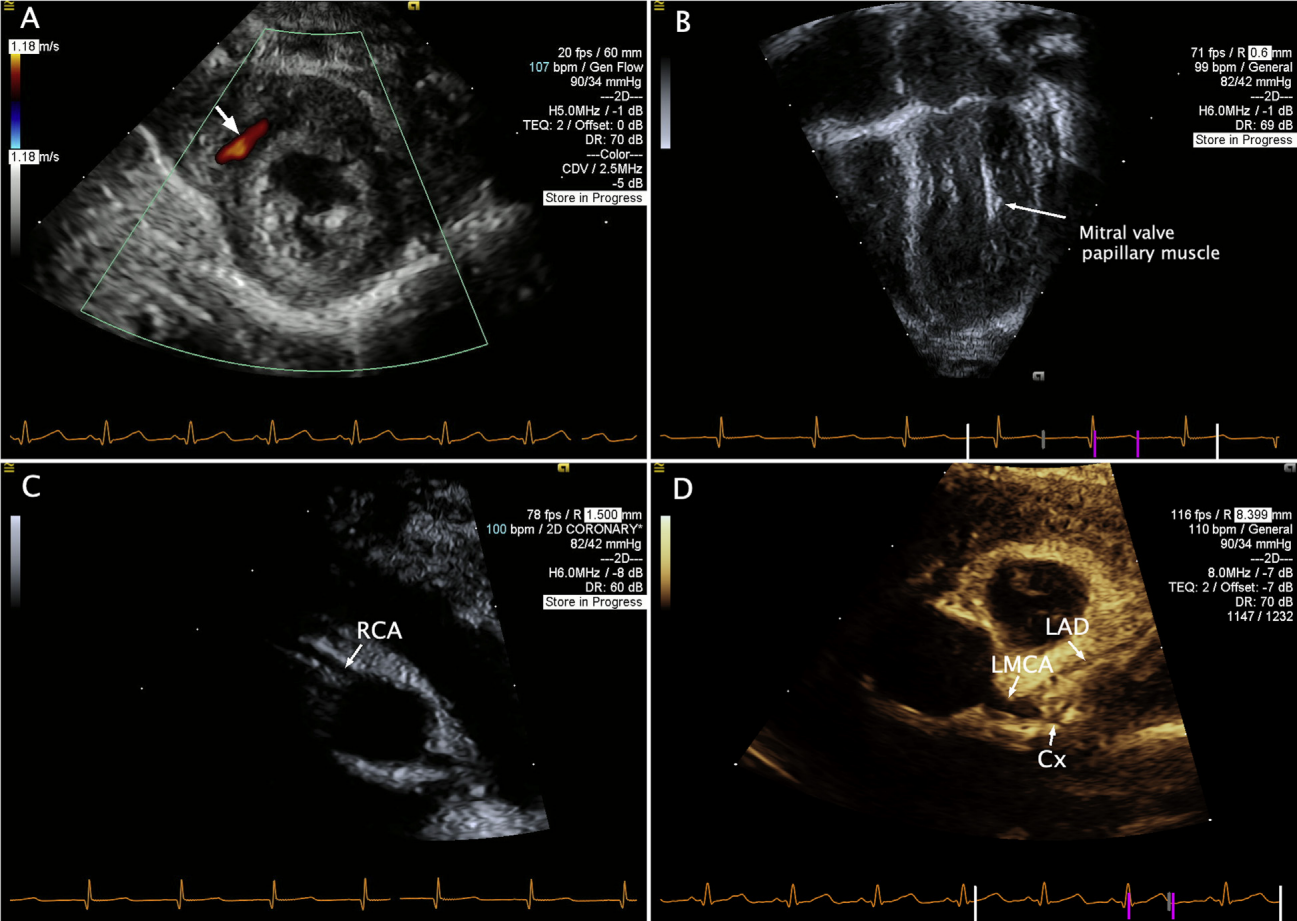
Transthoracic echocardiogram suggestive of coronary anomaly. Panel A demonstrates abnormal diastolic flow signal in the interventricular septum (*arrow*), and panel B shows an abnormally echobright mitral valve papillary muscle, both suspicious for a coronary artery anomaly. Panel C shows a normal RCA origin. Panel D demonstrates a normally positioned LMCA segment with normal branching of the LAD and circumflex (Cx) arteries. However, anterograde color flow could not be demonstrated by color Doppler imaging.
